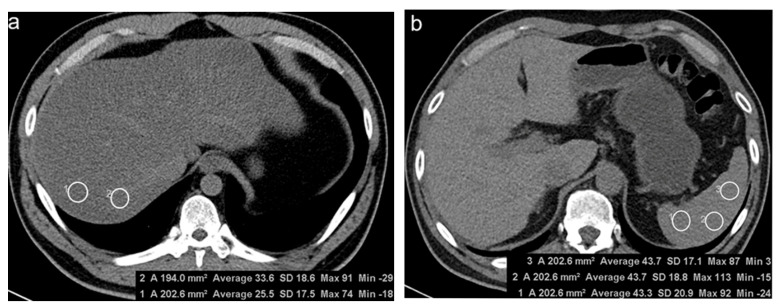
Measurement of hepatic (a) and splenic (b) attenuation on non-enhanced computed tomography. (a) Two 2 cm^2^ circular regions of interest (ROIs) were manually drawn at segment VII. (b) Three 2 cm^2^ ROIs were manually at three different sites in the middle third of the spleen.
